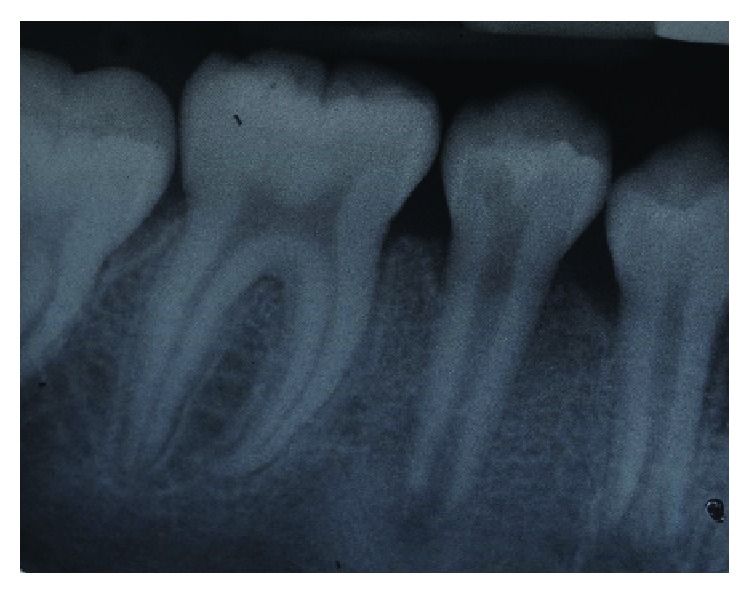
Periapical radiograph of tooth 45 showing immature root apex.
